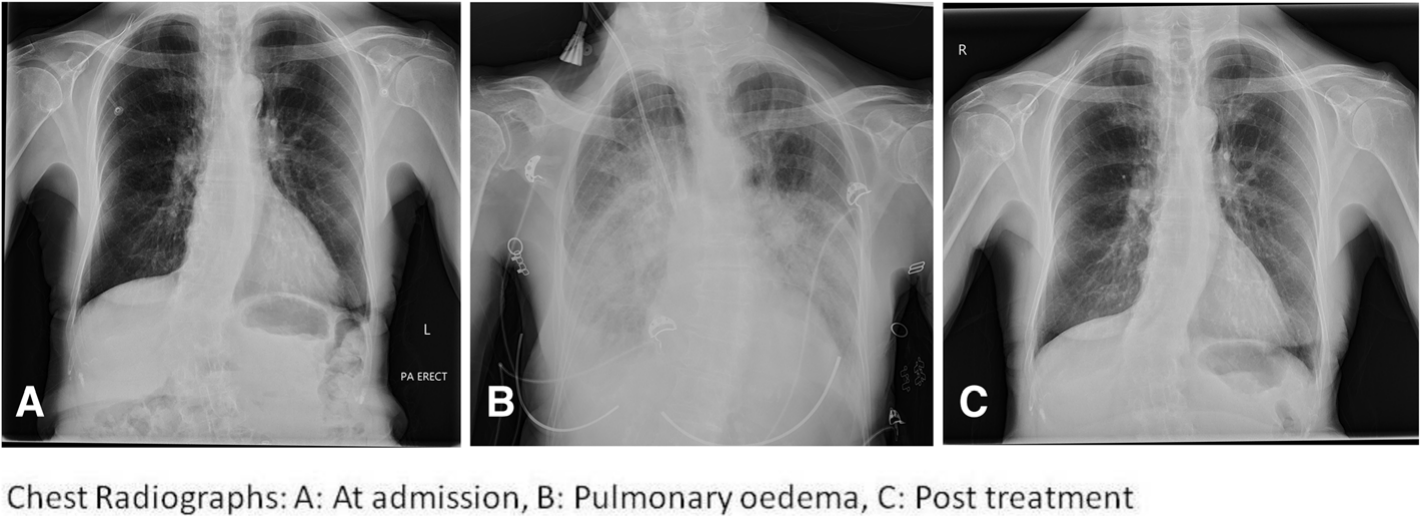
Chest Radiographs: A At admission, B Pulmonary oedema, C Post treatment
